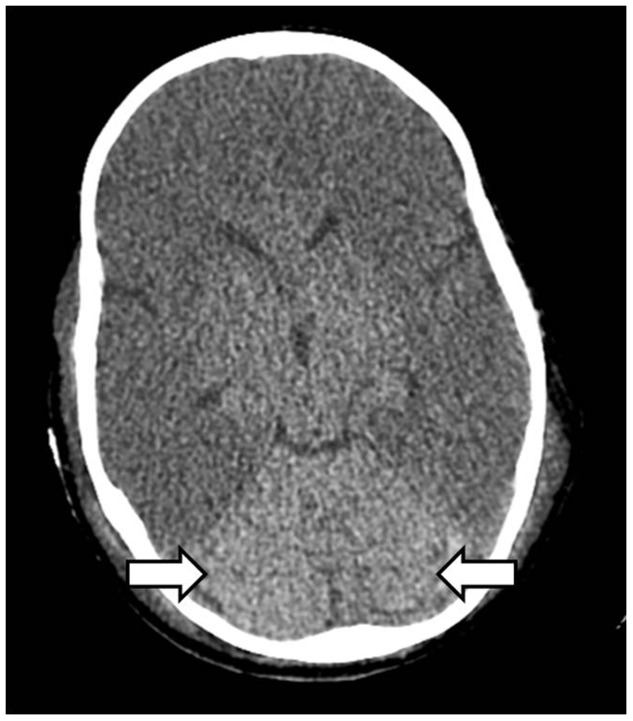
Cerebral edema. Axial non-contrast CT head showing diffuse hypodense cerebral parenchyma with loss of grey-white matter differentiation and classical white cerebellum sign (arrows).
